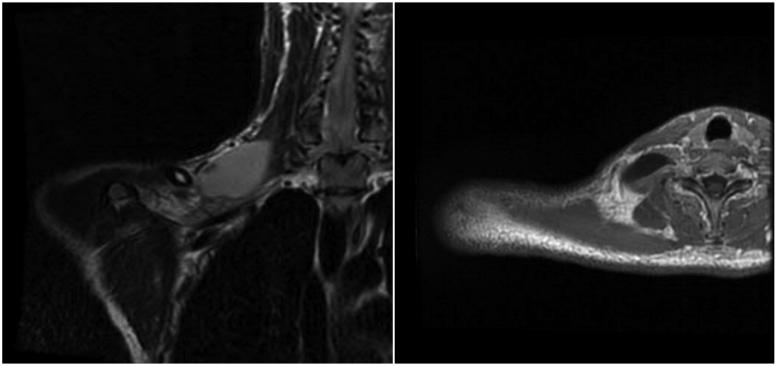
Patient’s magnetic resonance image showing a pseudomeningocele at C7 level. Coronal view on the left; axial view on the right.
